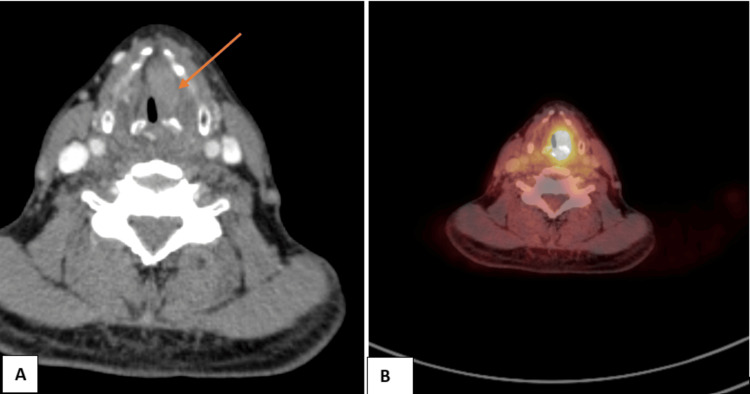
NI-RADS 2b follow-up PET-CT scan (A) Contrast-enhanced CT showing an increase in size of the previously ill-defined enhancing lesion in the left vocal cord, causing narrowing of the airway. (B) FDG PET scan of the same patient as mentioned in case 4 showed increased FDG uptake on a follow-up scan. Hence, it was upgraded to NI-RADS 3 on follow-up. FDG, fluorodeoxyglucose; NI-RADS, Neck Imaging Reporting and Data System.
